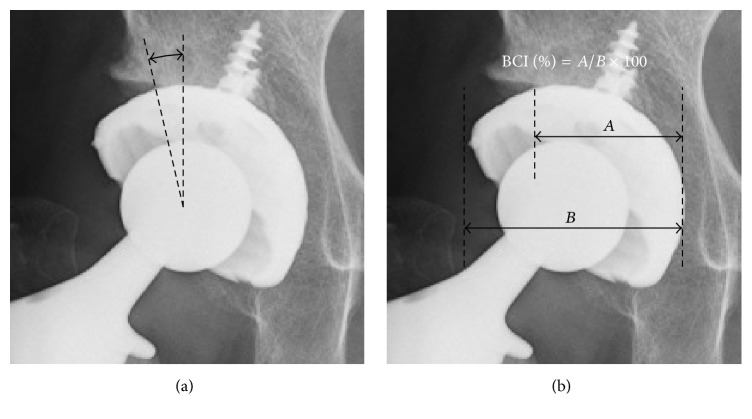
The cup center-edge (cup-CE) angle and bone coverage index (BCI) were determined on postoperative anteroposterior pelvic radiographs using the interteardrop line as a reference. (a) The cup-CE angle was determined as the angle created by the intersection of a line connecting the hip center and the lateral edge of the host bone and the line perpendicular to the interteardrop line. (b) The BCI was determined as the percentage of the horizontal width of the cup covered by the host bone (distance* A*) in the horizontal width of the cup (distance* B*).
